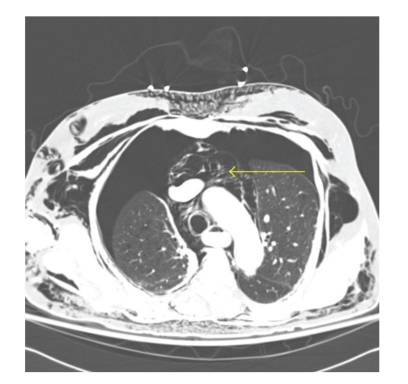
Transverse section of CT thorax showing subcutaneous emphysema, bilateral pneumothorax, and pneumomediastinum (arrow).
